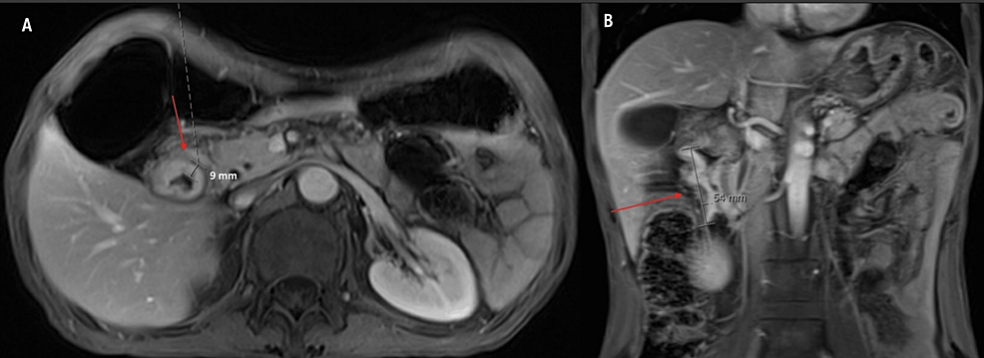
T1-weighted MRI with contrast. Cross-sectional T1-weighted MRI (A): Circumferential annular constricting duodenal wall thickening along the second duodenal segment, with the lesion measuring 9 mm in thickness. Coronal T1-weighted MRI (B): The lesion measures 54 mm in length and appears inseparable from the ampulla of Vater and the pancreatic head. MRI = magnetic resonance imaging
